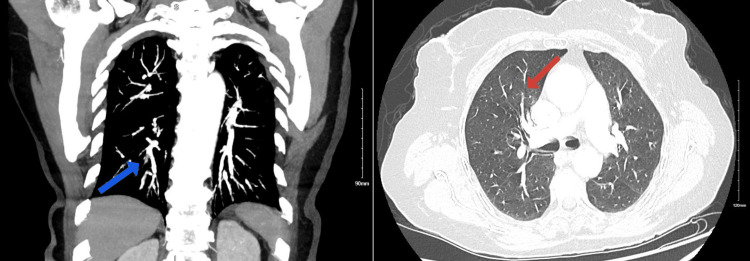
CT pulmonary angiogram showing filling defects in the subsegmental branch of the right lower lobe (blue arrow) and subsegmental branch of the right upper lobe (red arrow).
